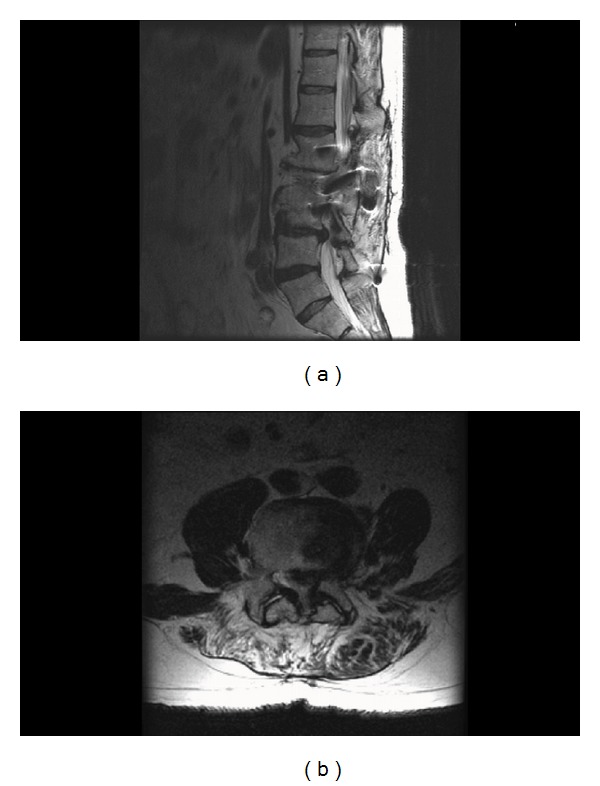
Sagittal (a) and axial (b) T2 weighted MRI scan of the lumbar spine displaying segment degeneration adjacent to a previous instrumented fusion with disc herniation as well as facet and ligamentum flavum hypertrophy.
